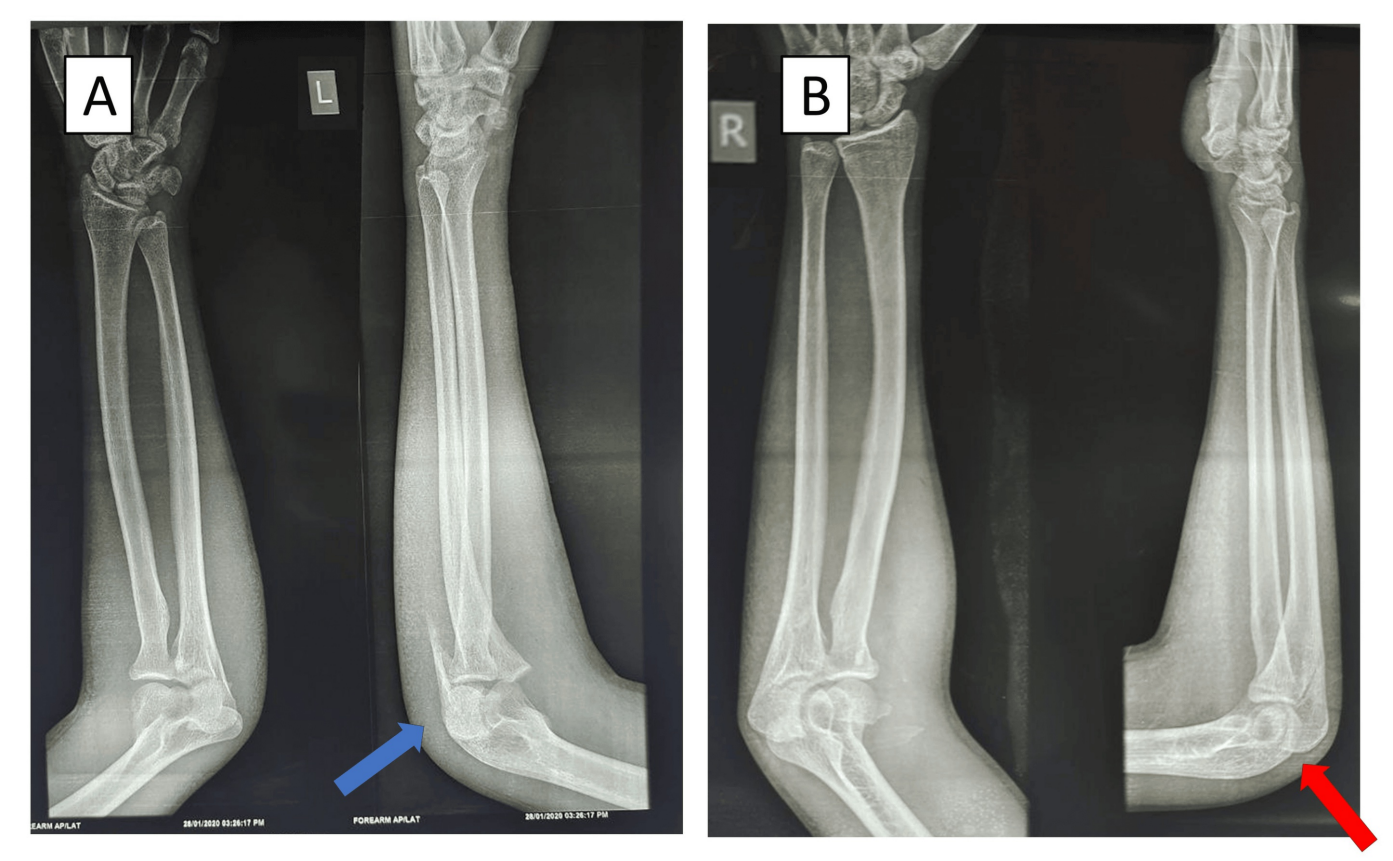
Preoperative radiographs of the left and right elbows The blue arrow shows a Mayo type II olecranon fracture on the left side, and the red arrow shows a Mayo type I olecranon fracture on the right side.
